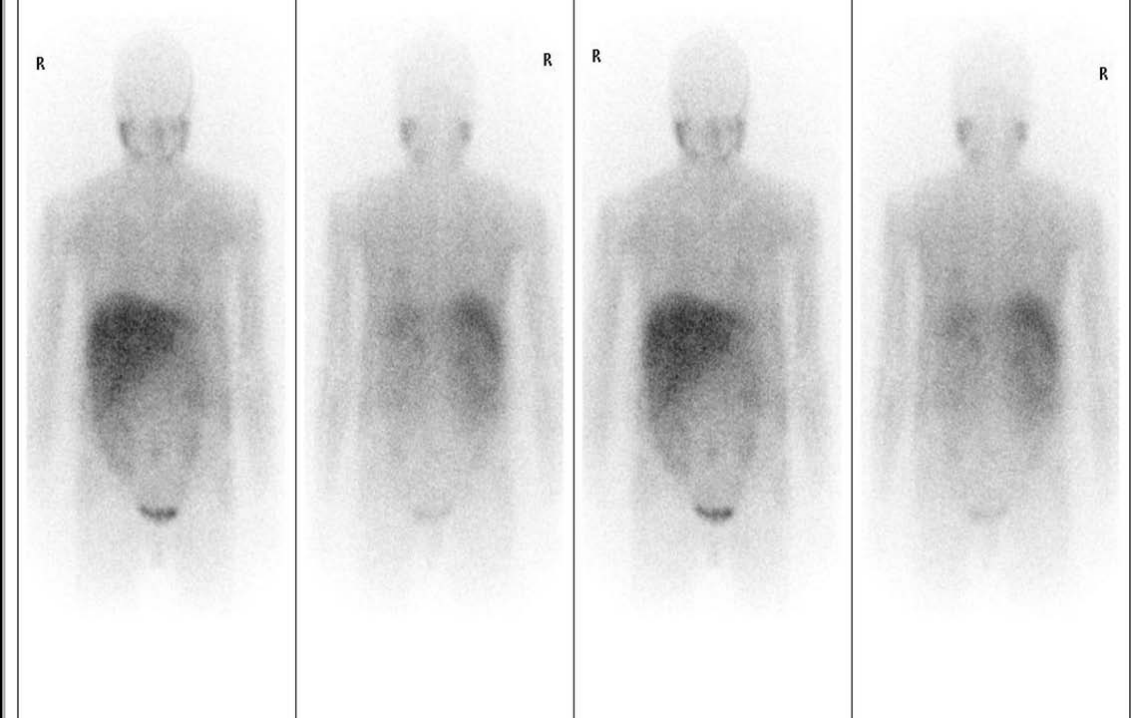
MIBG scintigraphy two weeks post-adrenalectomy displaying no evidence of residual chromaffin cell uptake or metastatic disease.
